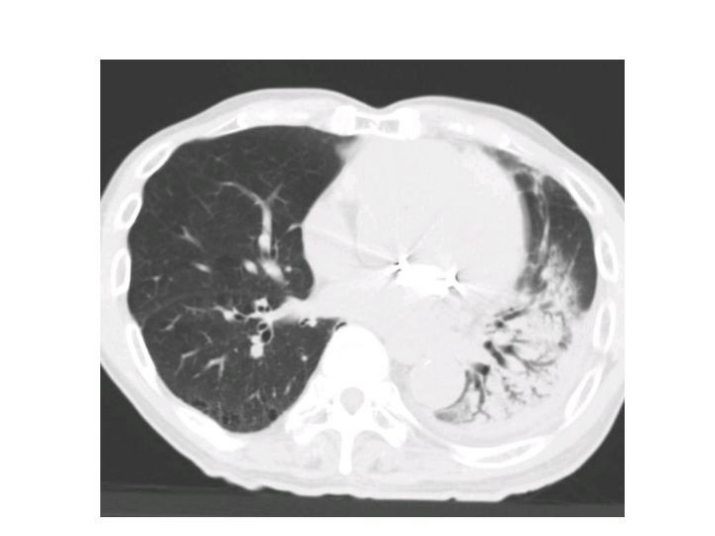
Computed tomography (CT) image of radiation pneumonitis (RP) (patient No. 11).
